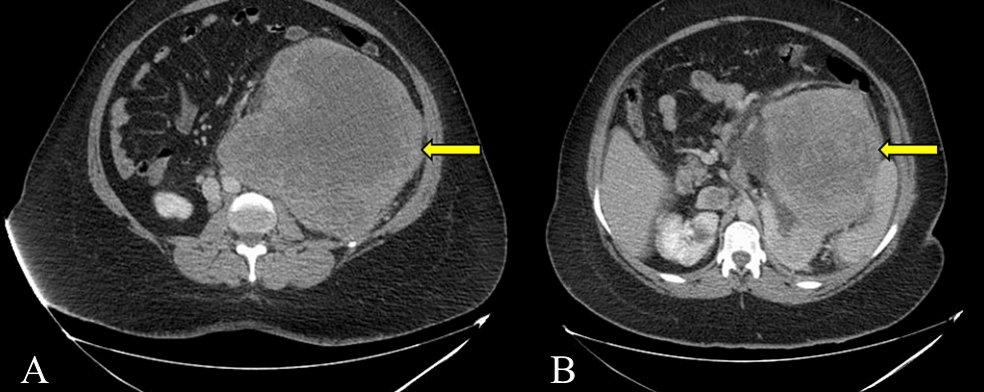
Axial CT image of primary Ewing sarcoma of the left kidney measuring 25 cm x 18 cm x 18 cm (arrows) CT: computed tomography
